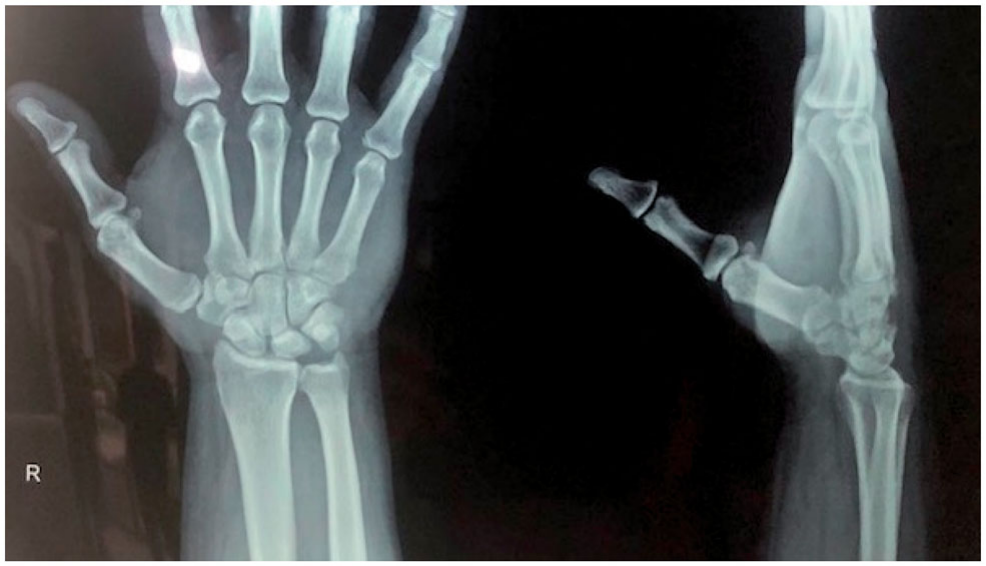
Follow up X-rays of wrist PA & lateral views CMC is in alignment.
